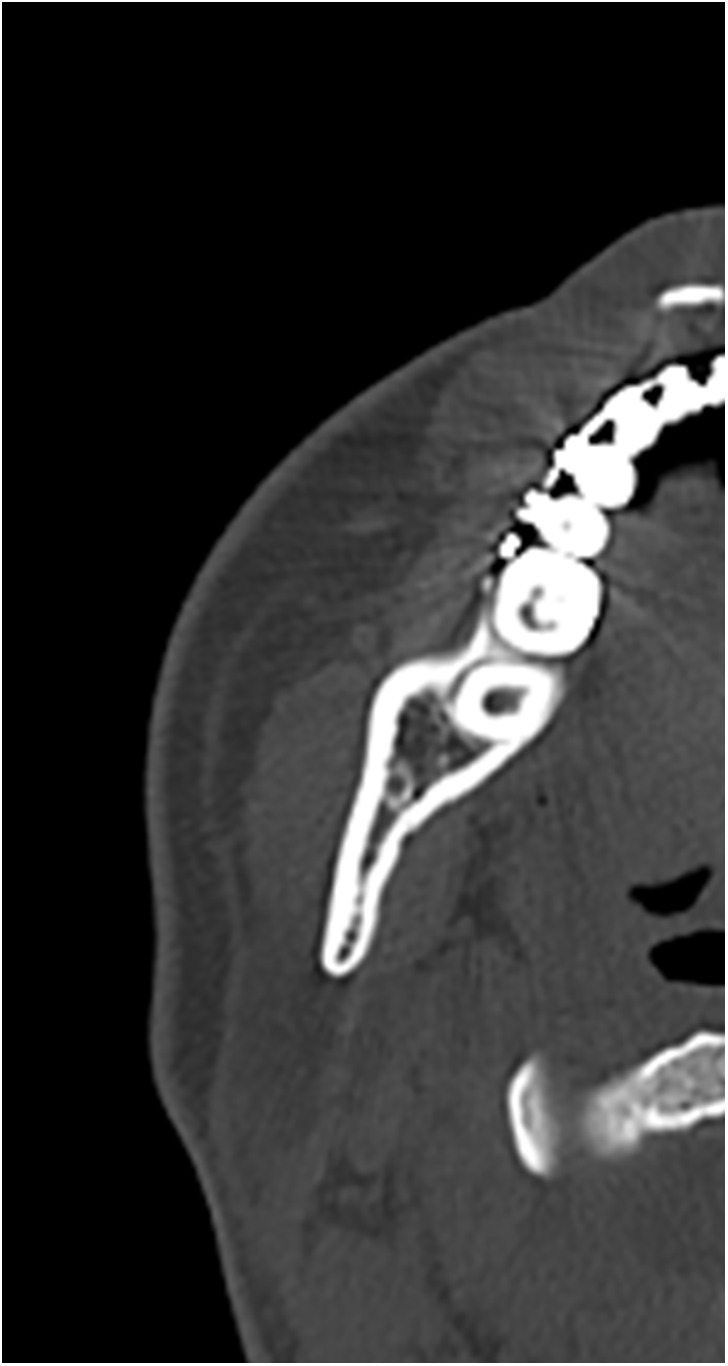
Axial computed tomography images showing contact between the mandibular canal and the lateral cortical bone.
